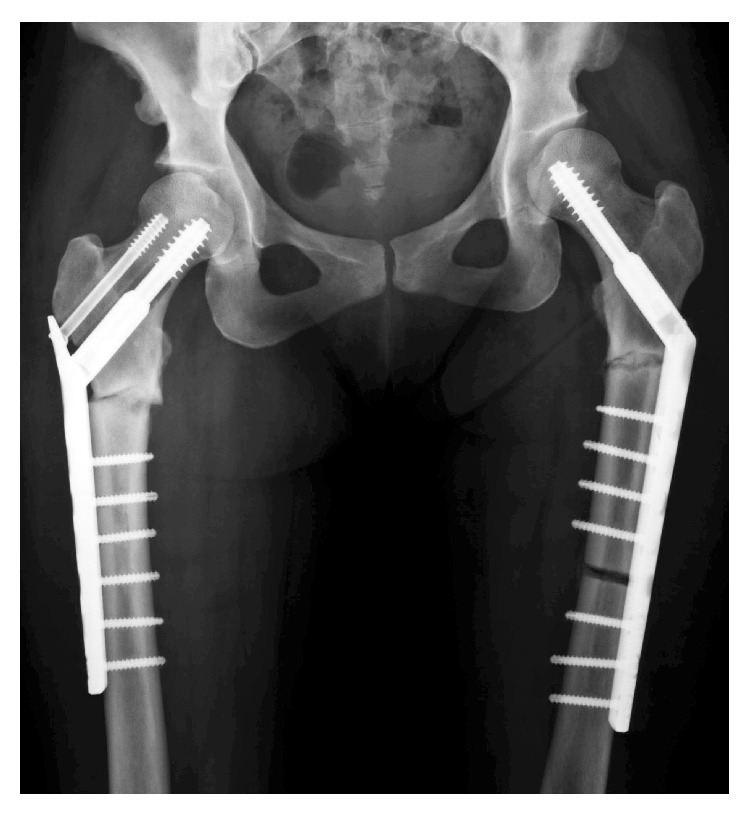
Radiograph after treatment of a fracture of the femoral shaft.
